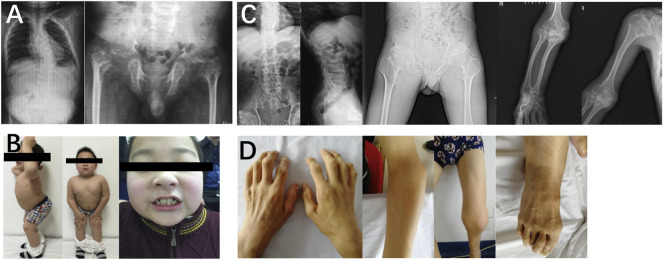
Photographs and radiographs of the families 3 and 4 with COL2A1-related skeletal dysplasia. (A,B) Radiographs of the spine and pelvis (A) and photographs of overall body and face (B) of proband 3 (Ⅱ-1) in family 3. (A) Scoliosis and platyspondyly were shown in the spine. Flattening and irregularity of the acetabular roof, femoral head necrosis, and disappearance of the femoral neck were visible in the pelvis. (B) Barrel chested and limited hip movement were performed in the overall body. No eruption of bilateral incisors was displayed in the face. (C,D) Radiographs of the spine, pelvis, and upper limb (C) and photographs of hands, elbows, knees, and feet (D) of proband 4 (Ⅱ-1) in family 4. (C) Severe multiple compressions of the vertebral bodies was revealed in the spine. Flattening of the acetabulum, shortening of the femoral neck, enlargement of the trochanter and femoral head, and narrow joint space of bilateral hips were shown in the pelvis. Widened epiphysis and metaphysis and joint cavity stenosis were visible in the elbows. (D) Bilateral elbow, knee, ankle, multiple metacarpophalangeal and interphalangeal joints were enlarged.
